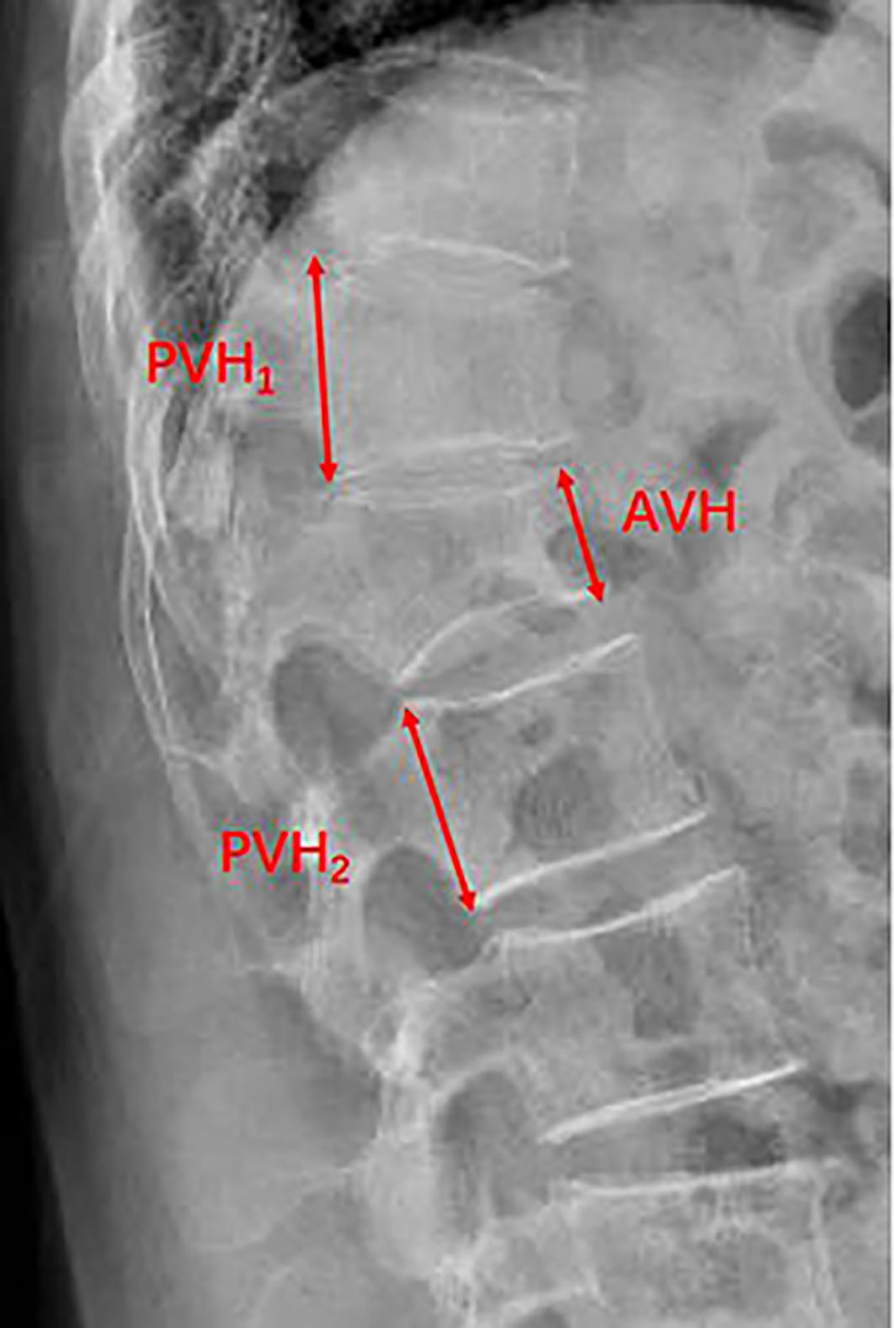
Plain radiograph showed the measurement of the anterior vertebral height (AVH) of the new fractured vertebral body, the posterior vertebral height (PVH_1_) of the adjacent cranial vertebral body, and the posterior vertebral height (PVH_2_) of the adjacent caudal vertebral body.
